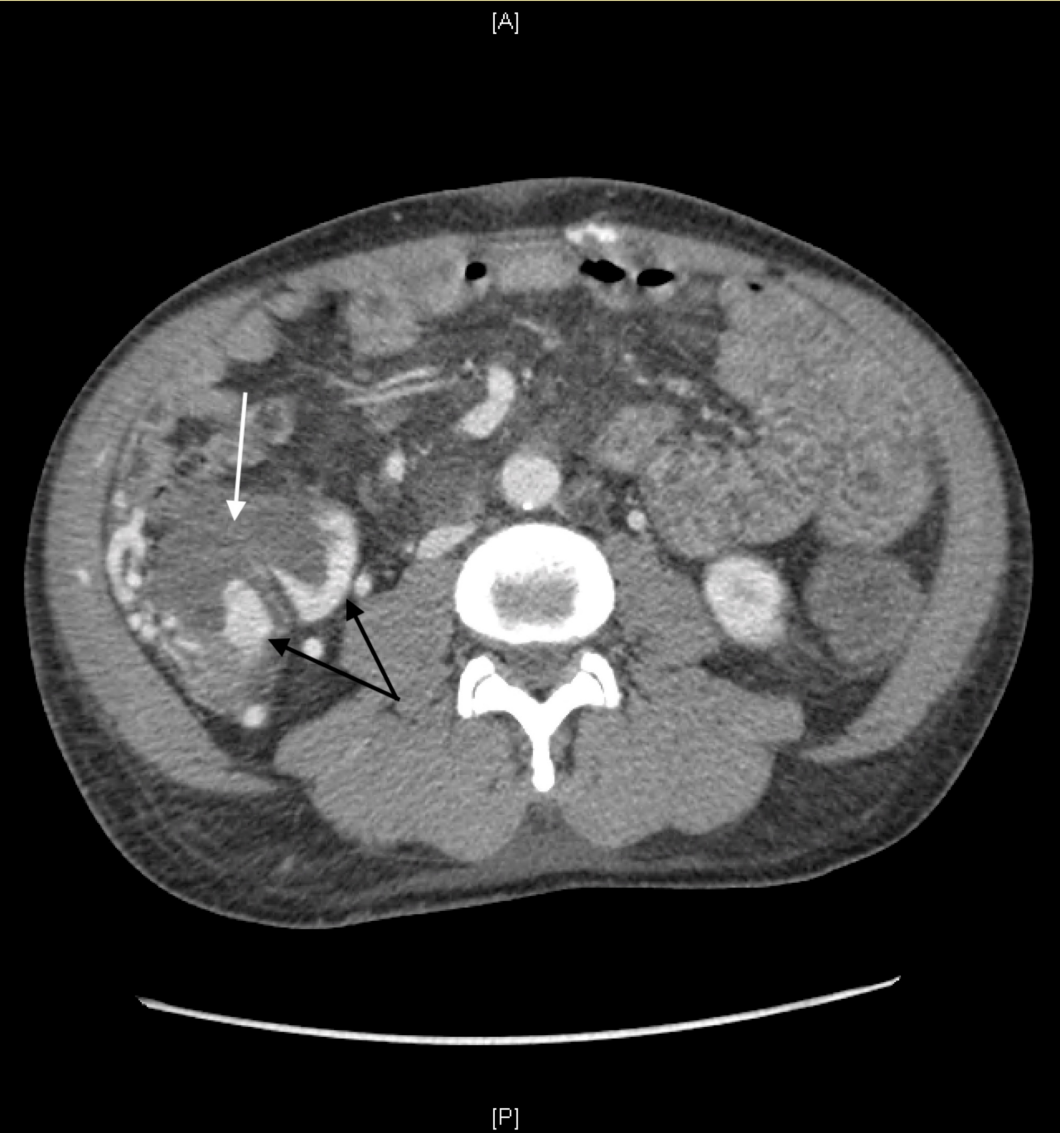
CT abdomen/pelvis with and without IV contrast. Pericecal varices (black arrows) in the right lower abdomen. Extravasation of iodinated contrast into a moderately distended cecum (white arrow) concerning for GI bleed.
